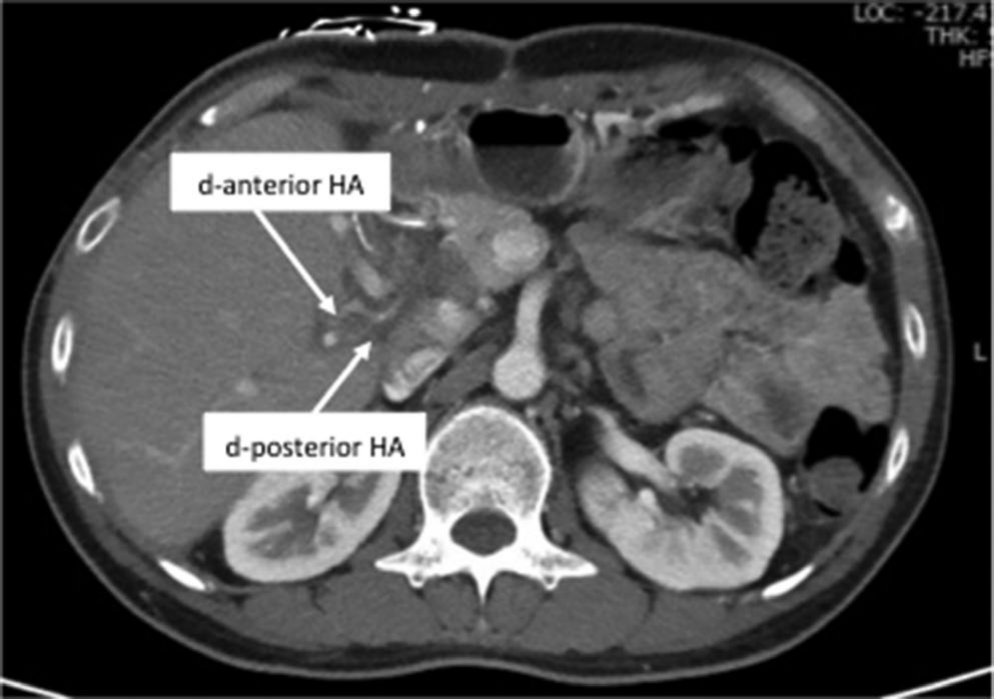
Postoperative CT images in Case 2. A, CT image of the reconstructed hepatic artery (anterior and posterior hepatic artery) on postoperative day 7
